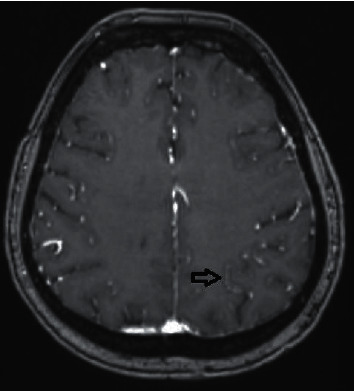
MRI from June 2012 showing hyperperfusion of the leptomeningeal arteries (black arrow).
